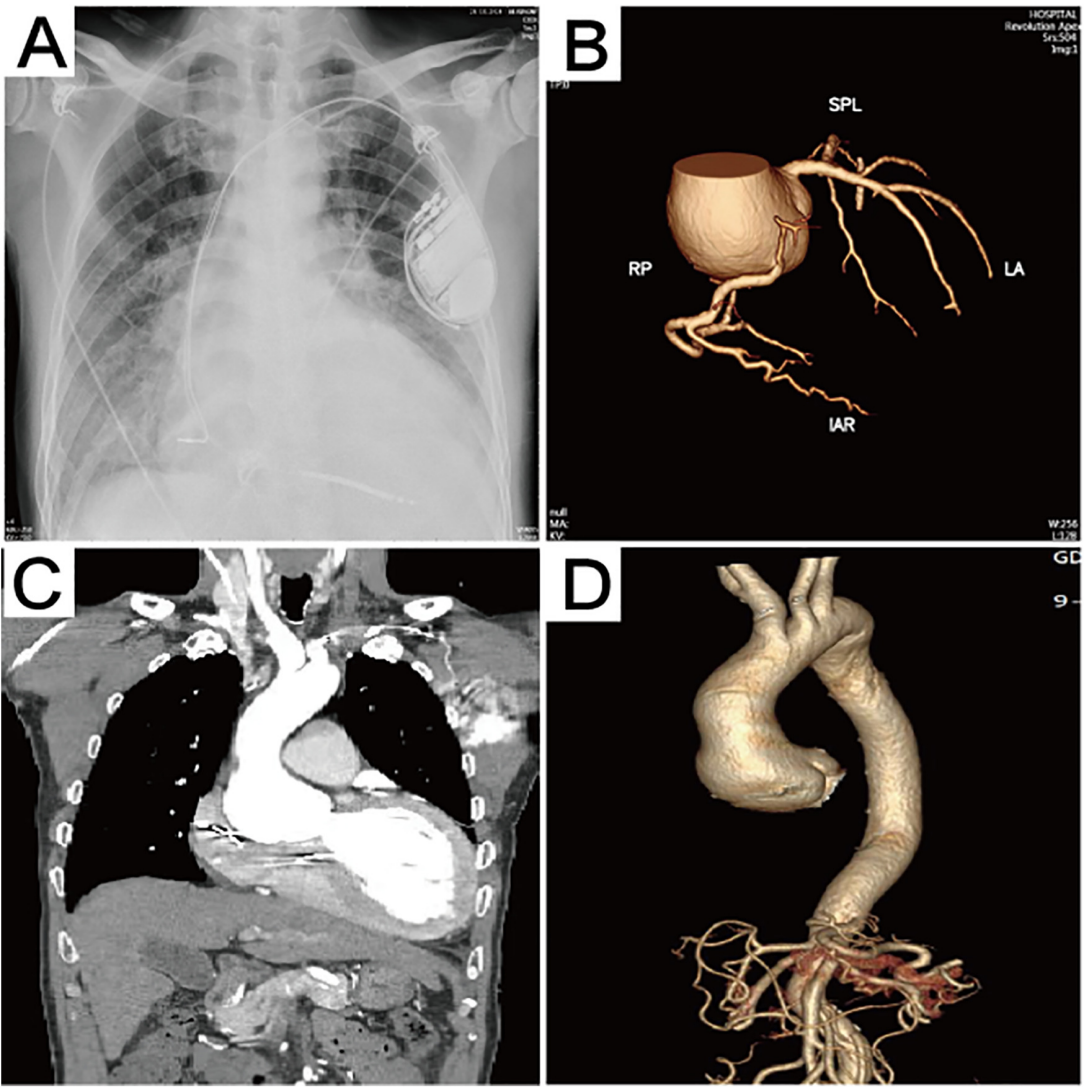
Preoperative imaging data. (A) The chest x-ray revealed the presence of pulmonary edema. (B) Computed tomography 3D reconstruction of the coronary arteries. (C) Preoperative computed tomography scan showing aortic sinus aneurysm, with the maximum aortic root diameter measuring 53.3 mm. (D) Computed tomography 3D reconstruction of the aorta.
